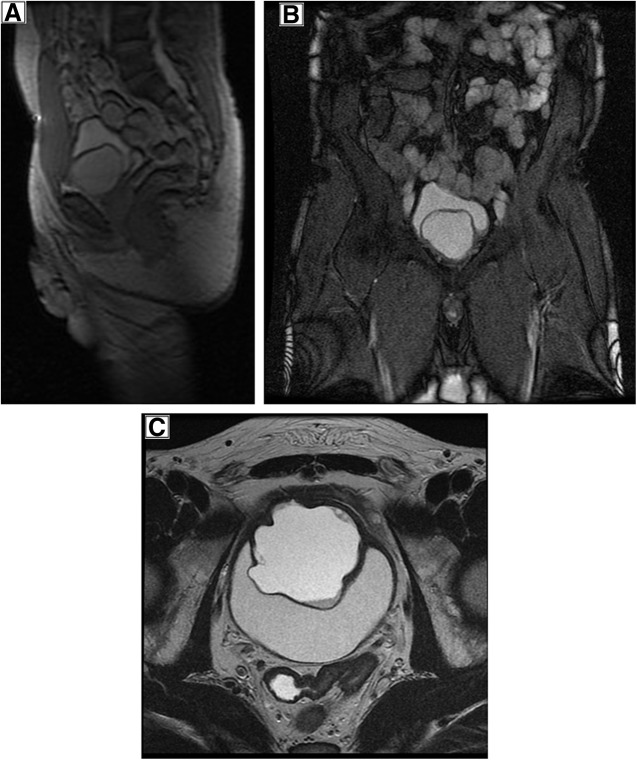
MRI pelvis/prostate showing the well-defined cystic lesion (A) 3pl LOC MRI; (B) Cor 2d Fiesta MRI; (C) T2 weighted ax FrFSE MRI scan.
